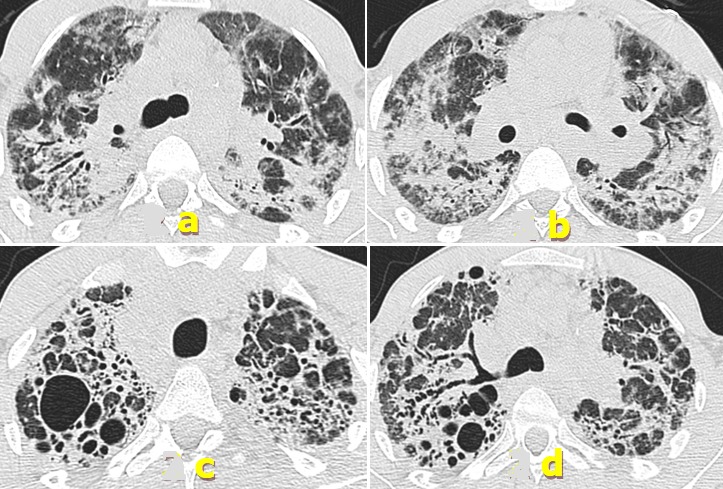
CT scan (lung windowing): a & b) bilateral lung consolidations and ground glass opacities with bronchial distortion; c & d) CT scan at 2 years: partial regression of lung consolidations and ground glass opacities and increase of distortion signs and cavities appearance
